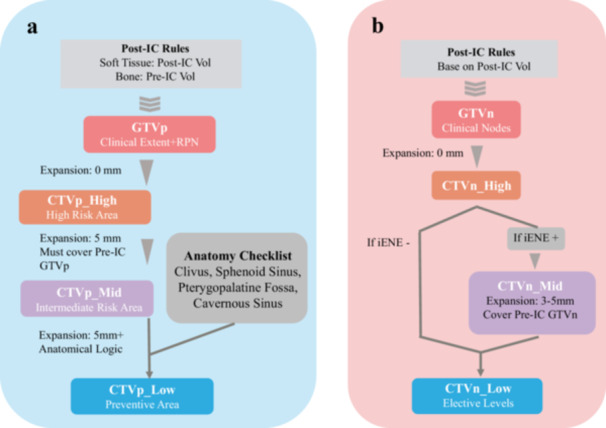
Consensus algorithm for target volume delineation in nasopharyngeal carcinoma (2025 update). (a) Strategy for the primary tumor. The GTVp is defined based on tissue response to induction chemotherapy (IC): soft tissue/mucosal targets are delineated on post‐IC MRI, whereas bone/sinus invasion retains the pre‐IC volume. For cavernous sinus involvement, the pre‐IC extent is included while excluding normal brain parenchyma. CTVp_Low requires specific anatomical inclusion based on T‐stage (e.g., delineating the anterior 1/3 of the clivus for T1‐2 vs. the whole clivus for T3‐4; excluding the sphenoid sinus upper half for T1‐2). (b) Strategy for neck nodes. CTVn_Low covers elective nodal stations, standardly including bilateral levels II, III, Va, and VIIa (lateral RPN), with level Ib included only for high‐risk cases. CTV, clinical target volume; GTV, gross tumor volume; IC, induction chemotherapy; MRI, magnetic resonance imaging; RPN, retropharyngeal nodes.
